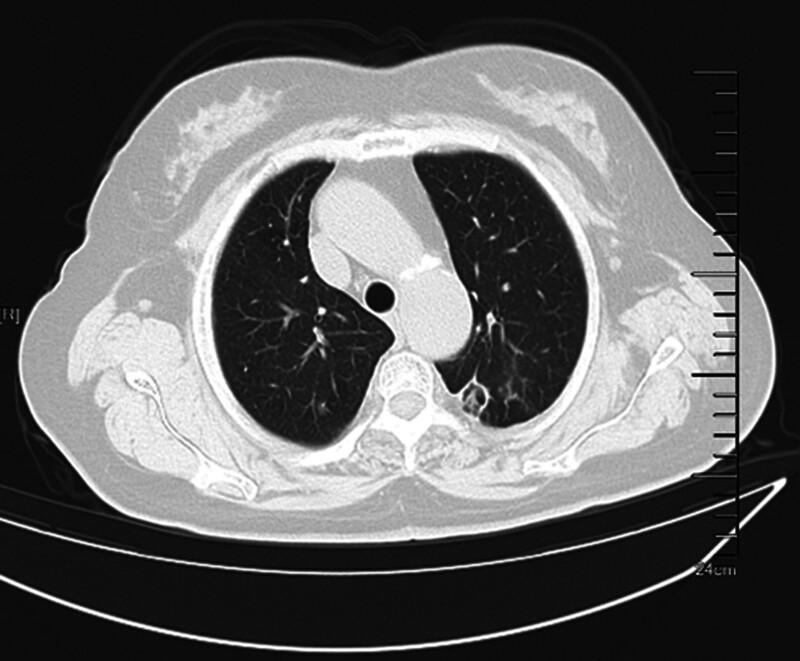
Lung window. A chest CT re-examination 9 days after oral voriconazole treatment (Figs. 6 and 7) showed that the lesion area was reduced. CT = computed tomography.
